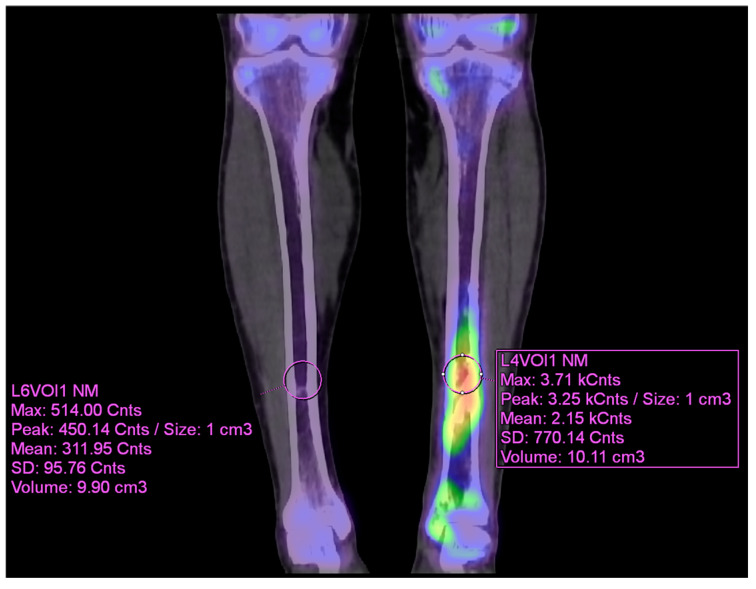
VOI (volume of interest) analysis of preoperative ^99m^Tc DPD bone SPECT/CT coronal image (contralateral side and affected side) using Syngo.via.
